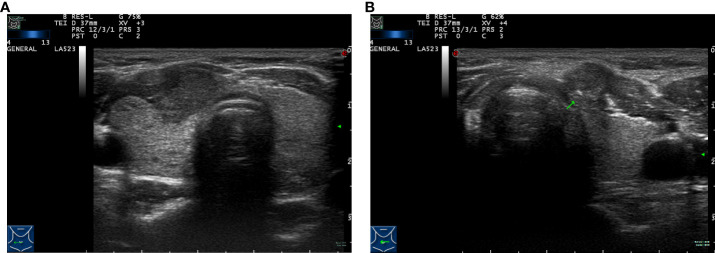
Preoperative ultrasound images of tumor lesions. (A) Capsule invasion. (B) Extrathyroidal extension (arrow).
